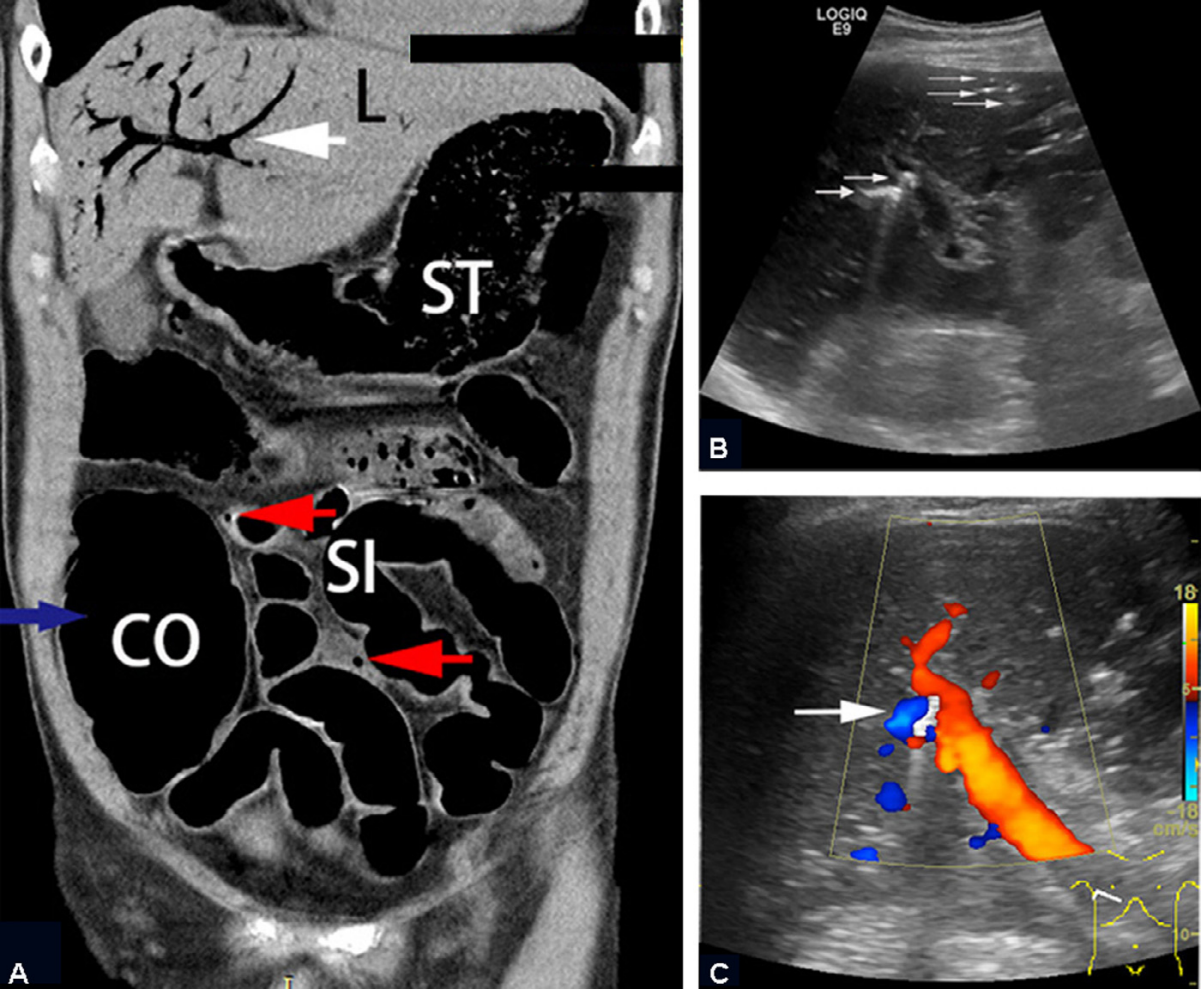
Imaging findings of PVG by CT and conventional US. (A) CT coronal view of liver with extensive hepatic PVG (white arrow), mesenteric vein gas (red arrow) and dilated colon (blue arrow). (B) Gray-scale US image showed scattered hyperechoic spots with “comet tails” in the hepatic periphery and a large volume of strong-echoic gas embolus in the right anterior branch of the portal vein (arrow). (C) Color Doppler US showed an artifact in the portal vein where gas embolus partially blocked the blood flow. (L, liver; ST, stomach; CO, colon; SI, small intestine)
